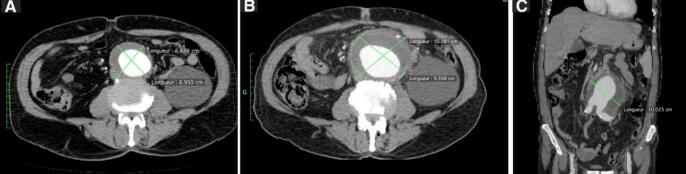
Computed tomography (CT) scan with contrast, axial section: demonstrates the aneurysm with no signs of inflammation (A), axial section:2 weeks later, a 3.4 cm growth with signs of periaortic inflammation (B) and a coronal section showing extension of the 10 cm mycotic aneurysm (C).
